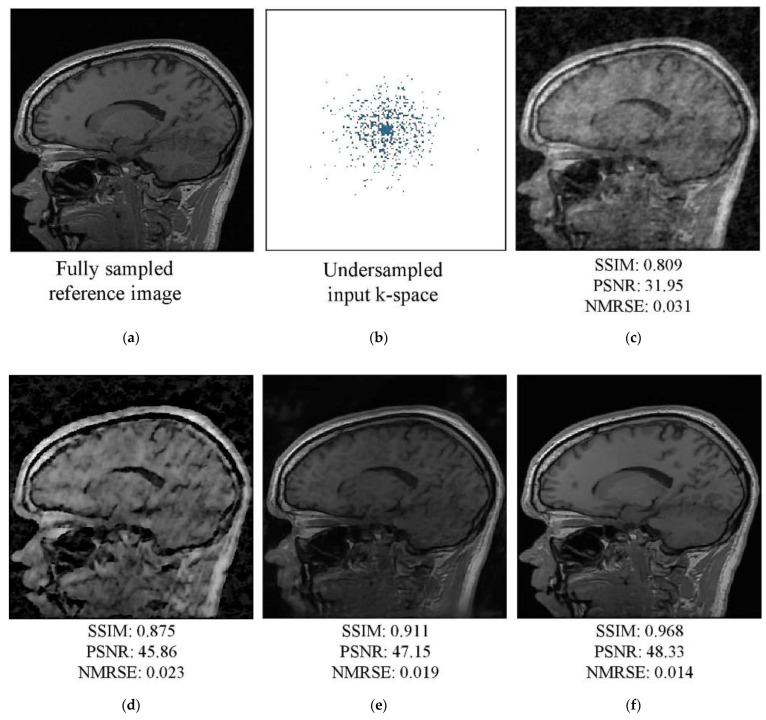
MRI images from the test dataset (slice No. 100) for AF 5: (a) fully sampled reference image and (b) undersampled k-space. Image reconstructed by the (c) IFFT, (d) Unet, (e) W-net, and (f) RA-CNN methods.
